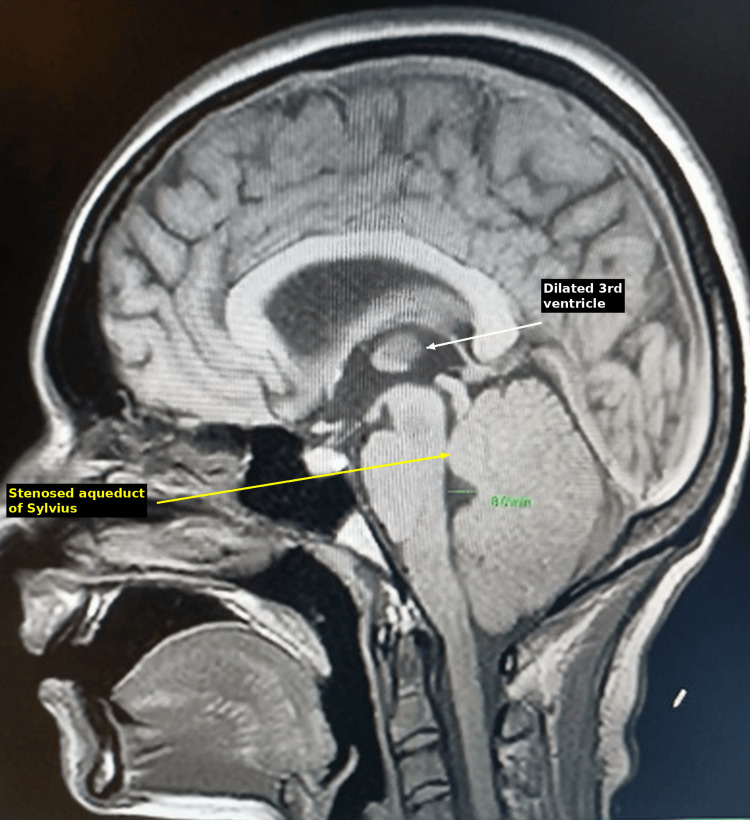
Sagittal T1-weighted MRI of the brain revealing stenosis of the aqueduct of Sylvius (yellow arrow), with associated dilation of the third ventricle (white arrow), suggestive of obstructive hydrocephalus MRI: magnetic resonance imaging
